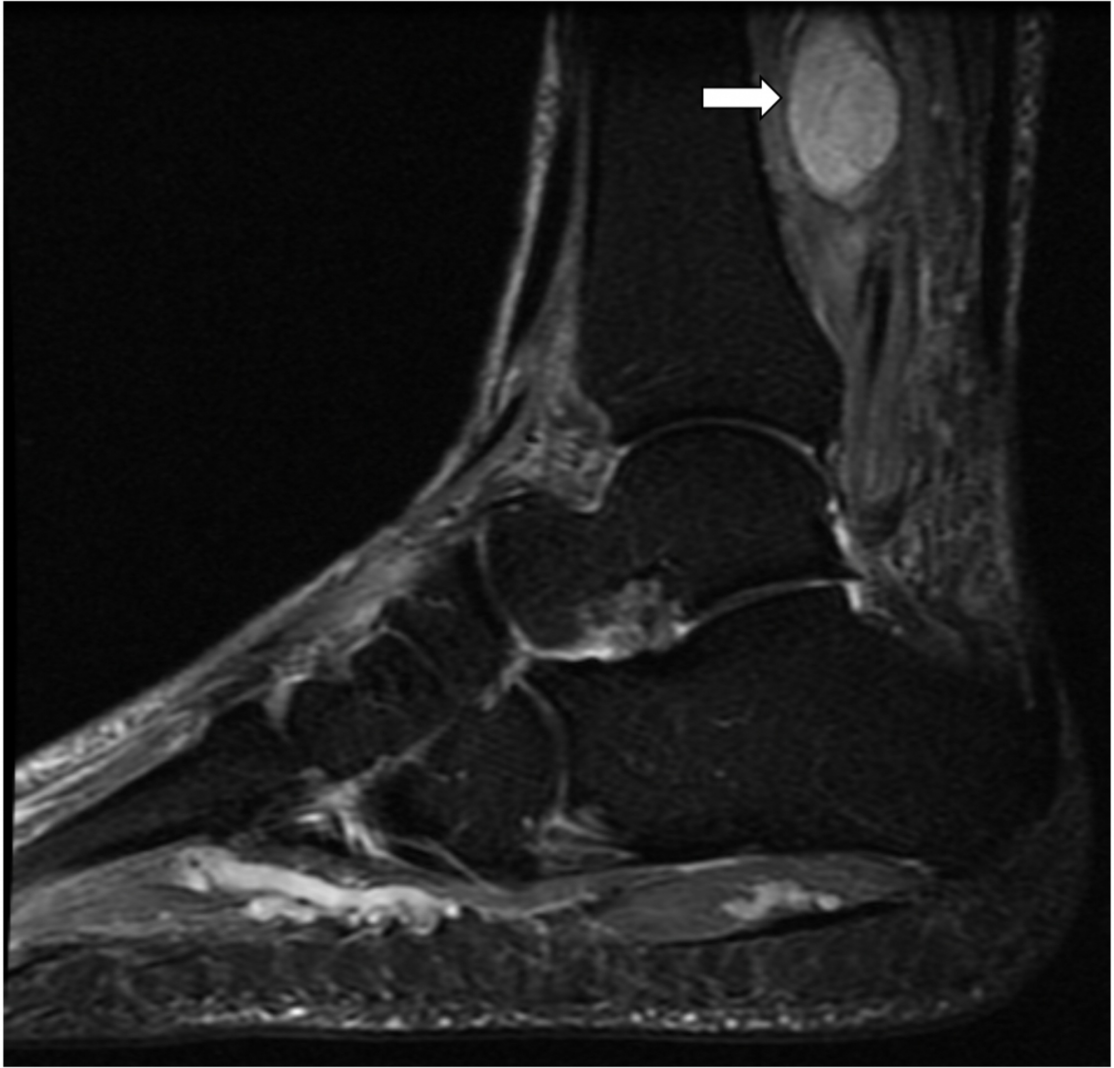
T2 weighted sagittal MRI showing the mass arising from posterior tibial nerve
